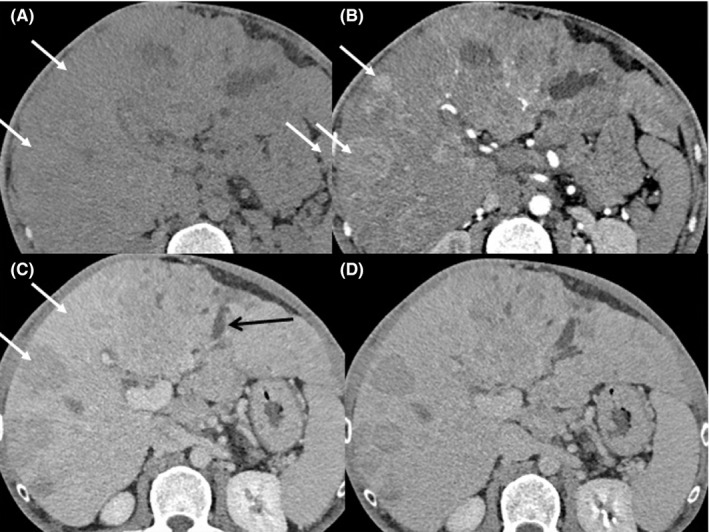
Multiphase abdominal computed tomography: The liver parenchyma is dysmorphic with irregular surface, portal vein enlargement and portosystemic collaterals. Numerous hepatic nodules and masses (White Arrow) isodense before contrast (A), enhancing vividly during arterial phase (B) and then washes out rapidly, becoming indistinct or hypoattenuating in the portal venous phase (C) with enhancing capsule in the late venous phase (D). They are associated with perfusion abnormality (Arrow head) due to arterioportal shunts: Multiple hepatocellular carcinoma
